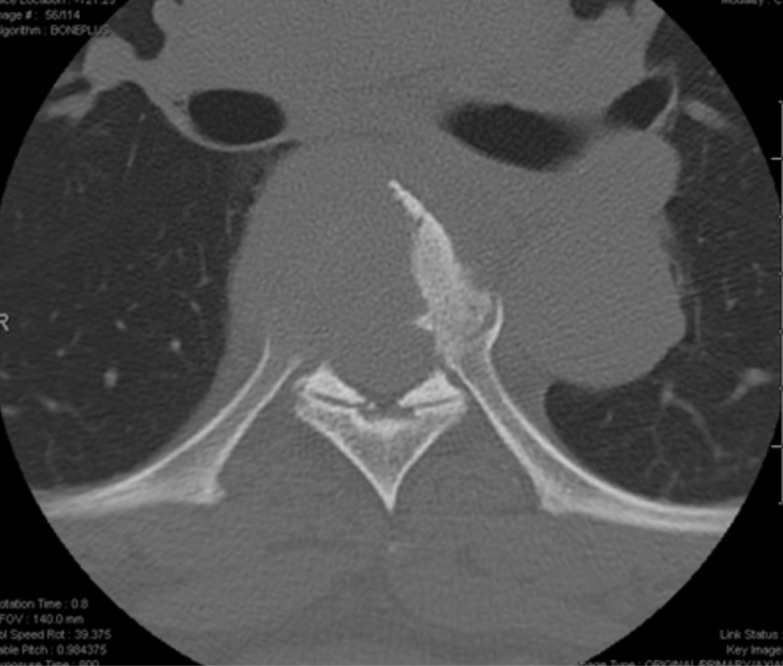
Axial CT of T7 Axial thoracic spine computed tomography (CT) demonstrating a lytic bone lesion at the level of T7.
